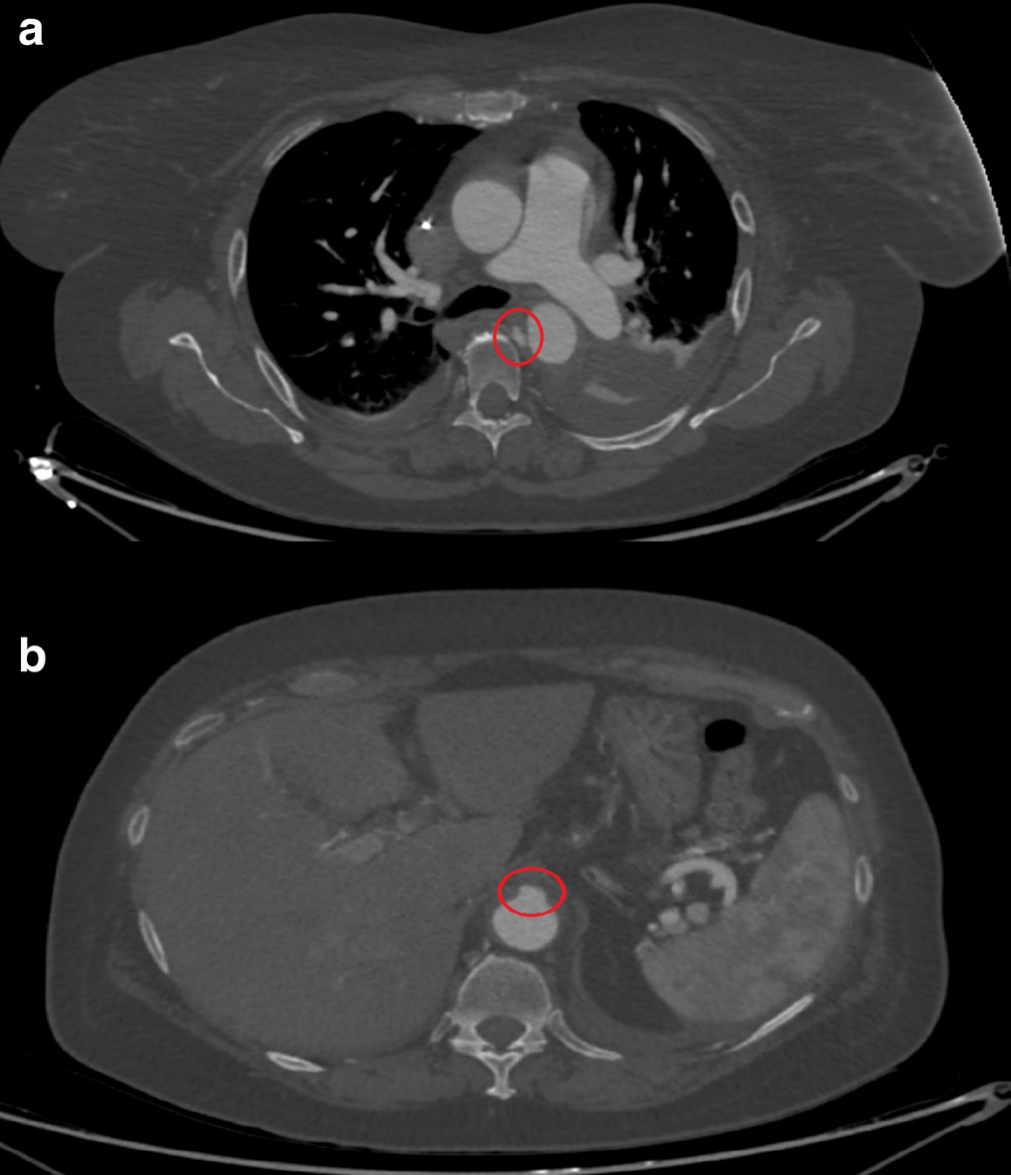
(day 8): Contrast-enhanced CT axial images at the level of the bifurcation
